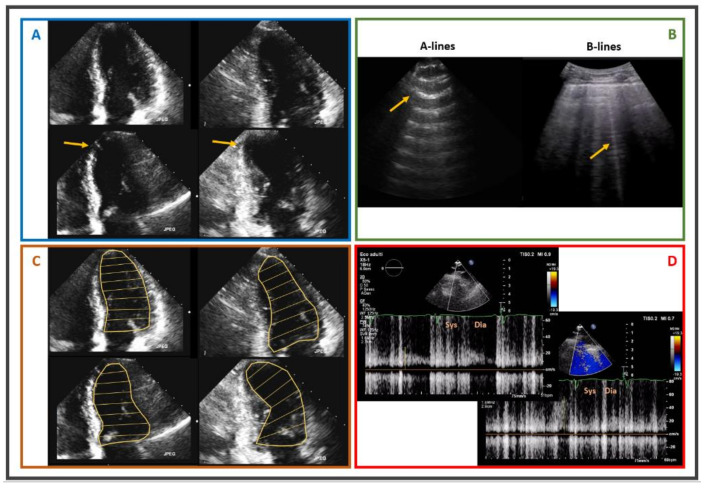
Exercise stress echocardiography performed in a 58-year-old male, smoker, with hypertension, who recently developed exertional chest pain. Panel (A): upper images show 4Ch and 2Ch views with normal kinesis at rest, while lower panels show 4Ch and 2Ch views under stress, characterised by apical septal akinesis. Panel (B): B-lines in lung ultrasound during stress. Panel (C): upper images show 4Ch and 2Ch views with normal LV size at rest, while lower panels show 4Ch and 2Ch views under stress, characterised by dilated end-systolic volume. Panel (D): blunted increase at peak stress of pulsed-wave Doppler diastolic flow at mid LAD. Patient was referred to ICA, pathological for critical stenosis of the mid LAD. *4Ch: four chambers; 2Ch: two chambers; LV: left ventricle; LAD: left anterior descending; ICA: invasive coronary angiography; Sys: systolic; Dia: diastolic.*
